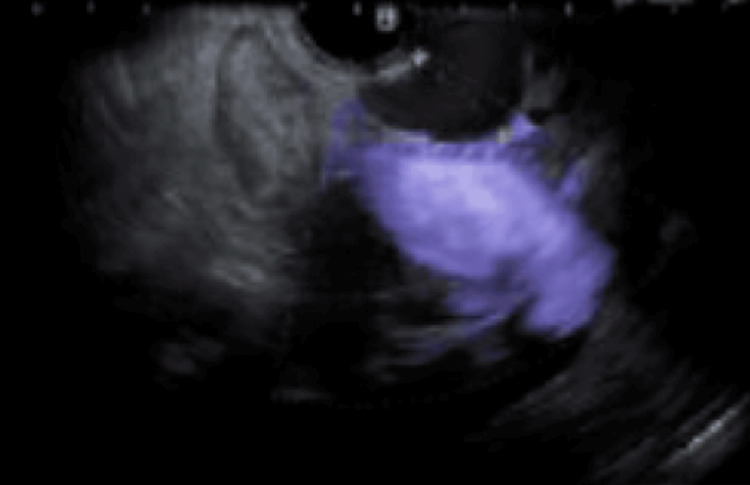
Endoscopic ultrasound demonstrating a hypoechoic ampullary mass arising at the level of the major papilla, measuring approximately 22.9 × 10 mm. The lesion appears confined to the mucosal/superficial layer without clear evidence of deep invasion into adjacent structures.
